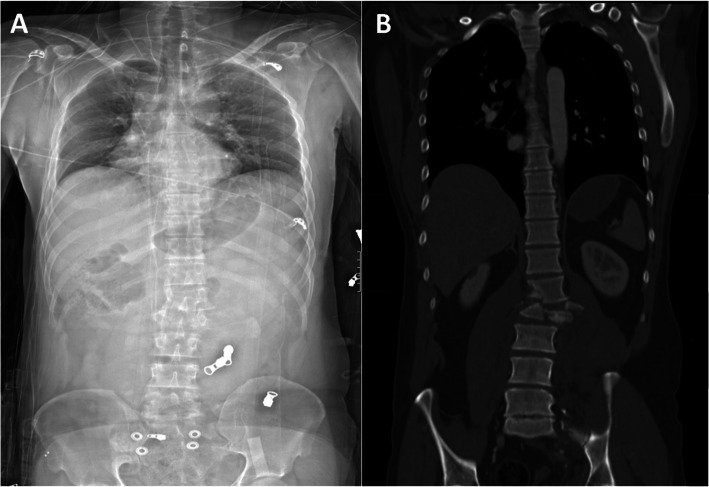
Example of a correctly identified unstable injury using anterior-posterior Lodox Statscan (AP-LS). C-type injury of the 12th thoracic and first lumbar vertebra shown in AP-LS (a) and the corresponding full-body CT scan (b) of 36-year-old men after a car accident. This injury was correctly identified by two of the three observers (Radiologist and Orthopedic attending)
